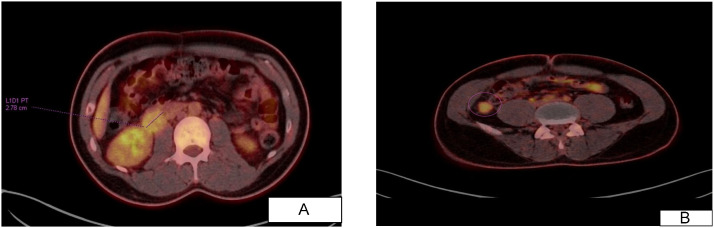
(A, B) – 18F-FDG PET/CT shows no evidence of hypermetabolic disease recurrence at the surgical bed, with a moderately hypermetabolic soft tissue lesion (SUVmax: 3.9) at the right renal hilar level abutting the right psoas muscle. Also, a few mildly to moderately hypermetabolic soft tissue nodules at the right paracolic gutter inferior to the right kidney, some of them abutting the right psoas muscle, the largest measures 1.4 × 1.7 cm with an SUVmax of 3.7.
